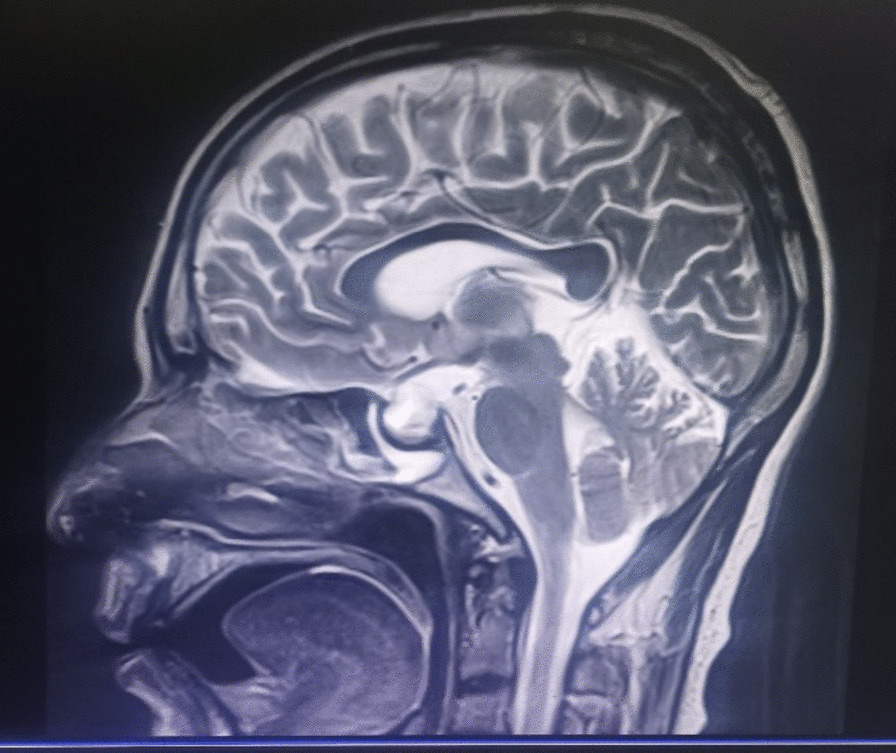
MRI of the brain/Sagital section
